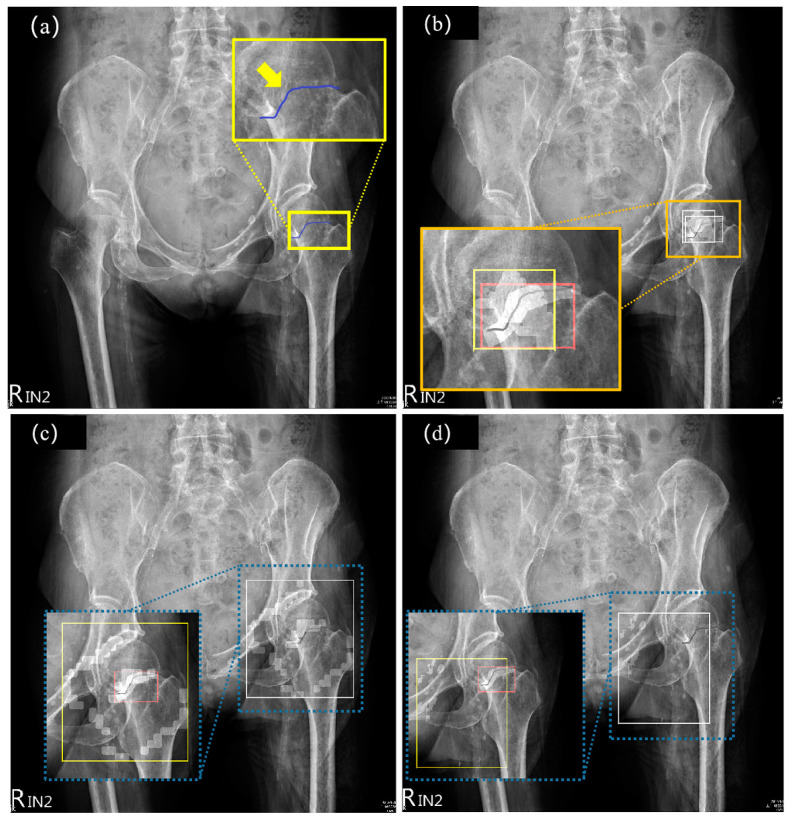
Radiographic images of pelvis and FNFs and the detection results of U-Net, DenseNet, and our proposed DAFDNet. The arrow indicates the location of the FNF and the dashed line indicates the zoomed-in area. (a) Imaging of the pelvis with FNF labeled by blue lines in the magnified view. (b) Fracture detected by DAFDNet, (c) fracture detected by U-Net, and (d) fracture location detected by DenseNet. As shown in the enlarged view, the fracture-detection range for each method is in the yellow rectangle and the physician-delineated labels are in the red rectangle.
